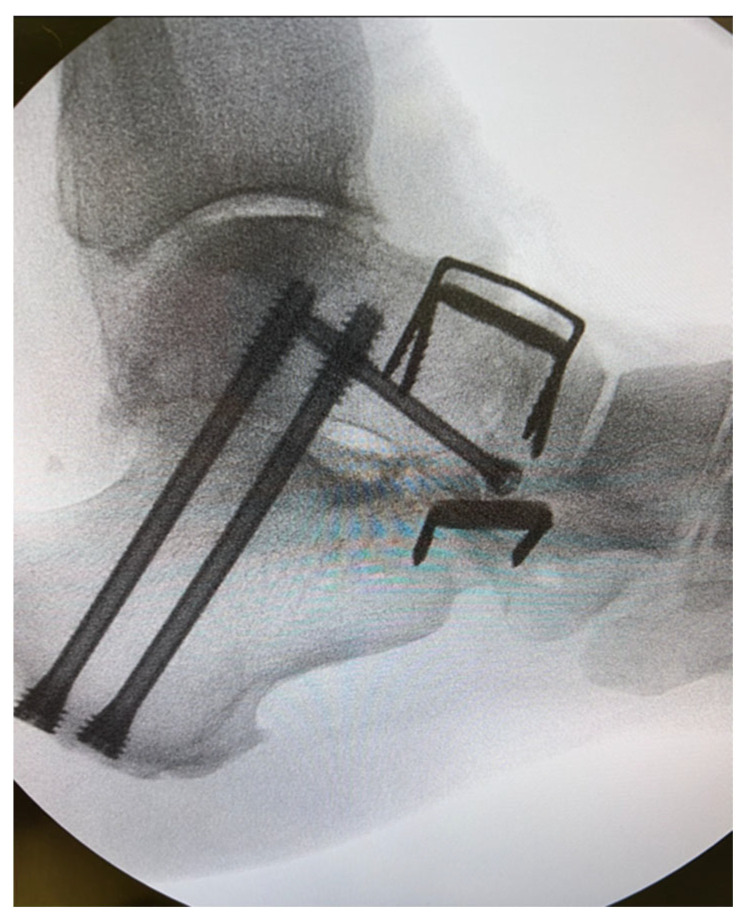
Lateral intra-operative radiograph depicting a realignment triple fusion performed with a combination of internal fixation techniques including CCIs. Note that the dorsal osteophytes at the talonavicular joint were debrided to allow for appropriate staple position, thus preventing anterior ankle impingement.
